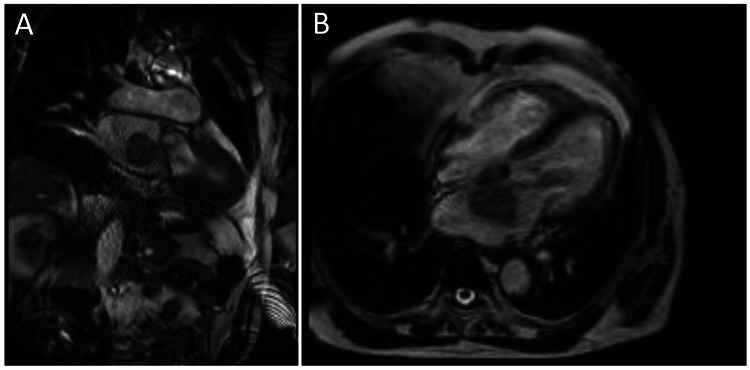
Cardiac MRI showing (A) 4.2 × 3.5 × 3.6 cm heterogeneous mass in the left atrium (LA) arising from the interatrial septum, with characteristics consistent with myxoma. (B) Mass is seen with an attached finger-like projection prolapsing through the mitral valve.
